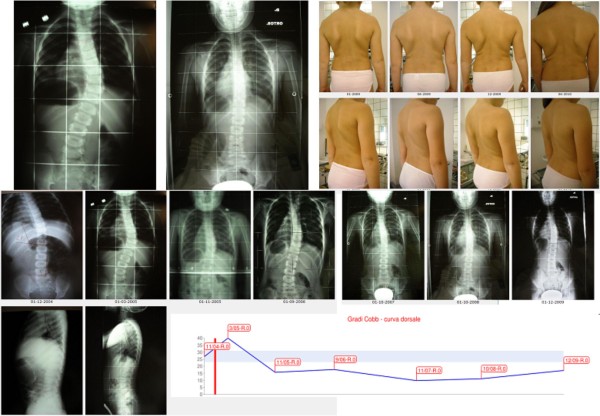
Fifth case report: infantile thoracic scoliosis patient of 45° who weaned the brace but is still in treatment. First line, left to right: x-rays at brace wearing (March 2005) and at brace weaning (October 2008), frontal and sagittal aesthetics in the last evaluations after brace weaning. Second line: all frontal x-rays of this case report of a patient still in treatment. Third line: sagittal x-rays, and the graph with obtained results. B.C. infantile thoracic right scoliosis was discovered at the age of 13 months, and rapidly progressed without treatment from 27° to 40° in 4 months as soon as she reached the standing position. A Sibilla brace treatment was then started 23 hours per day per 4 months and 20 during the summer, with an immediate reduction to 16° in 8 months. Brace was then gradually reduced 2 hours every six months while maintaining correction, and finally weaned with the curve at 10°. At the age of 6, as soon as was possible, everyday SEAS exercises (20' per session) have been started and are the only actual treatment.
